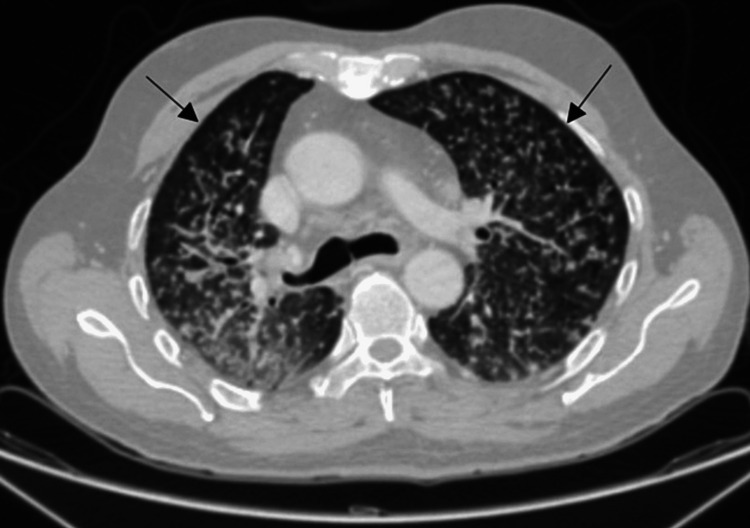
The chest CT with contrast, axial view (lung window), depicted multiple centri-lobular tree in bud nodulations. CT: Computed Tomography
